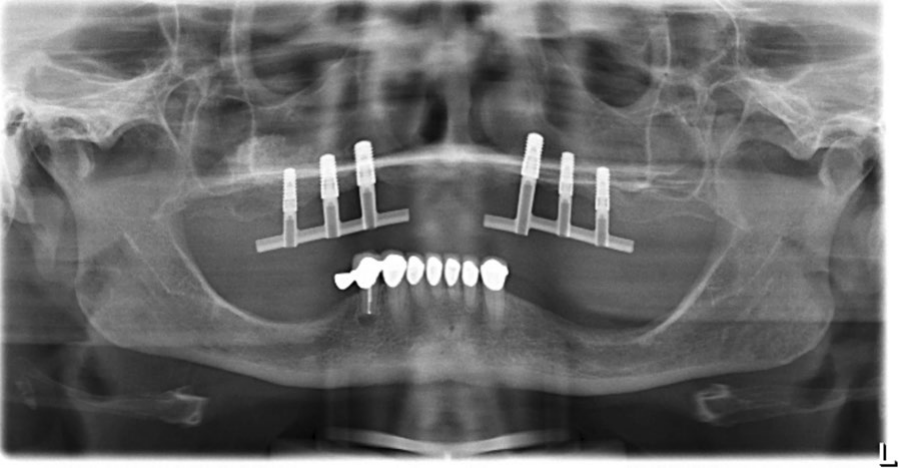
Panoramic X-ray of a bar-retained superstructure on six implants in the augmented maxilla (test: left side, control: right side)
